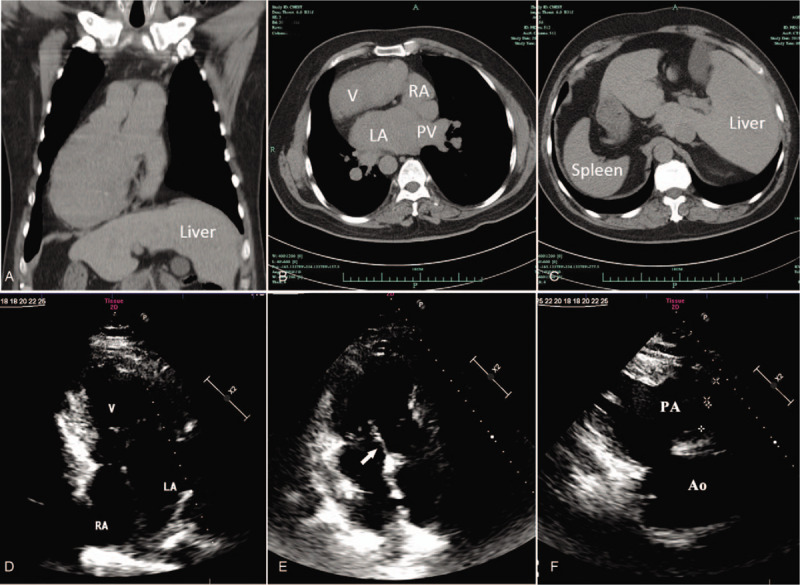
The images of computed tomography and transthoracic echocardiography of this patient. (A-C) CT showing heart in the right hemithorax with situs inversus and dilated single ventricle. (D-E) Echocardiography from apical 4-chamber view showing univentricular malformation with 2 normal sized left atrium and lengthy leaflets of tricuspid valve (white arrow). (F) Echocardiography from parasternal long-axis scan demonstrating both aorta (Ao) and pulmonary artery (PA) arises from single ventricle.
